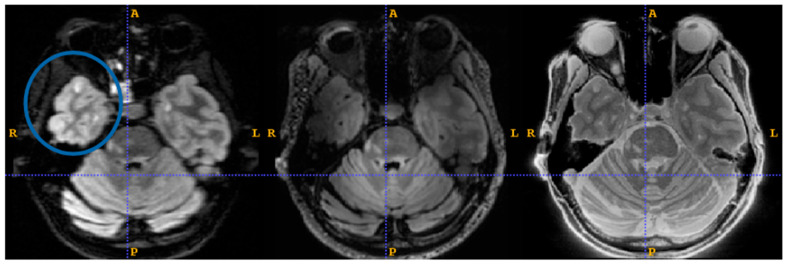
7T FLAIR contrast loss in left temporal lobe. MS lesions are visible (blue circle) on 1.5T FLAIR (left) and SilentMT (right). MS lesions are not visible on 7T FLAIR (centre).
